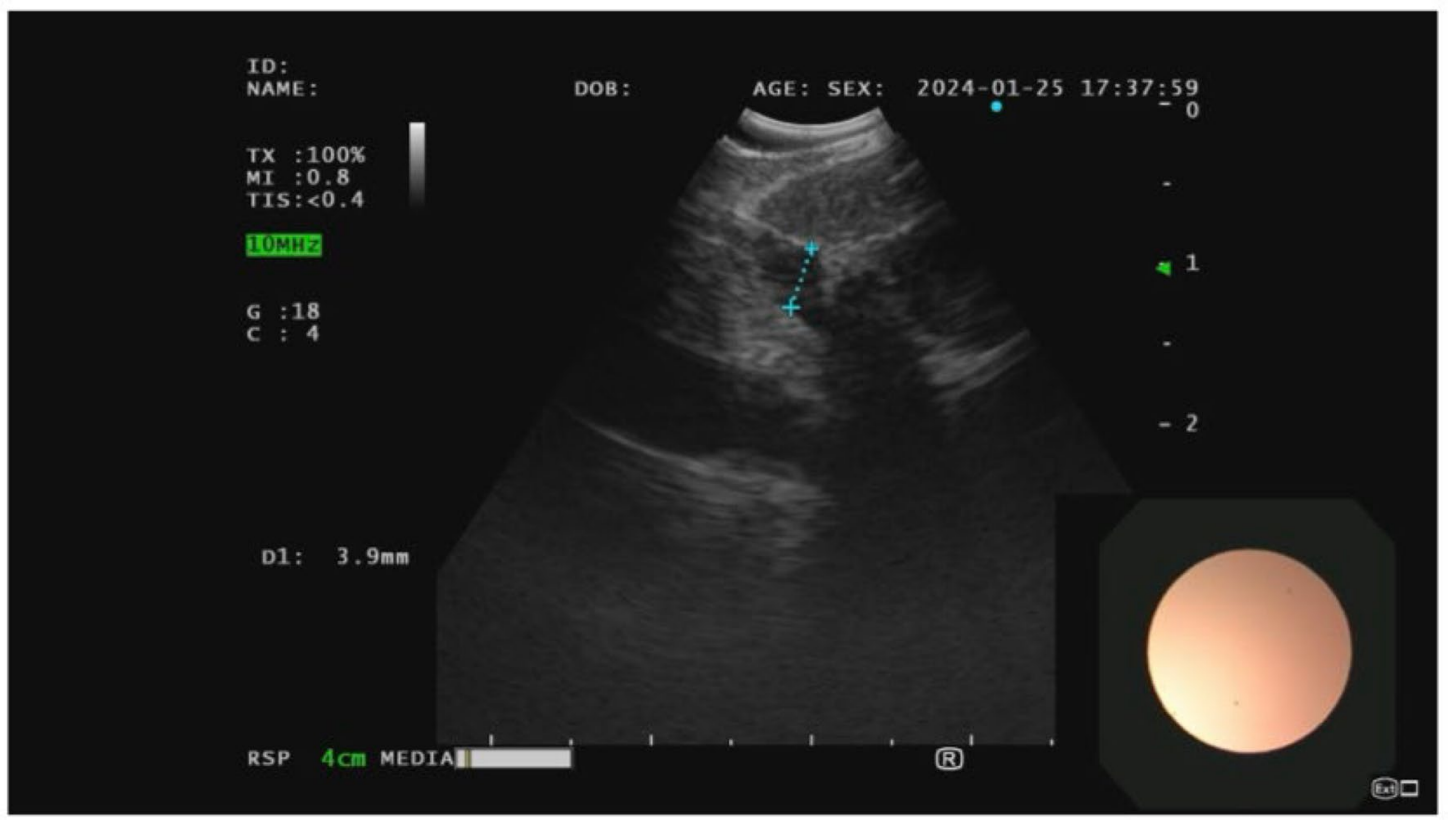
Linear endobronchial ultrasound of the paratracheal soft tissue nodule
